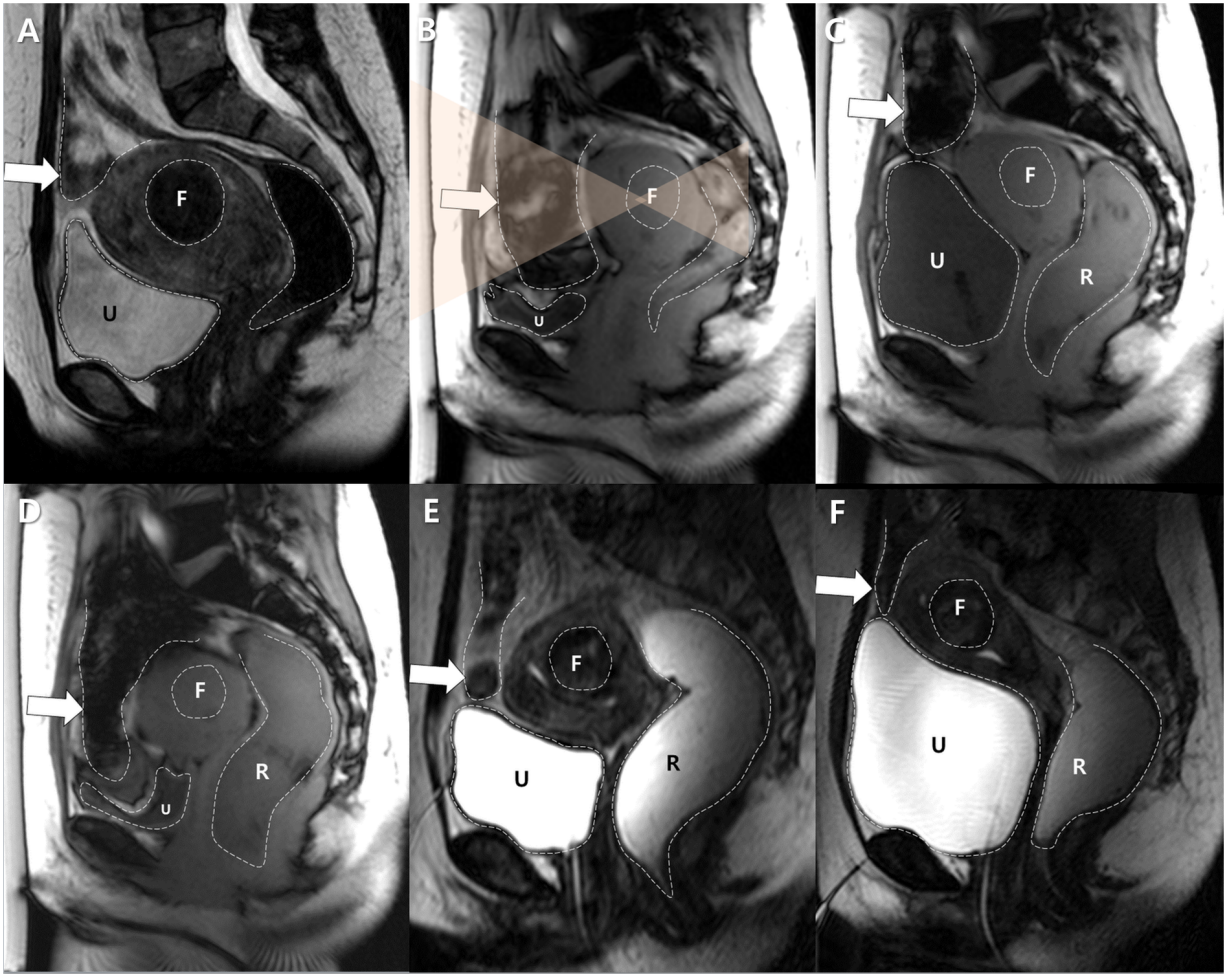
A case of technical failure of the BRB maneuver in a 42 year-old woman with a fibroid in a forward-bent uterus. The patient did not undergo GnRH agonist pretreatment. A. Sagittal image of screening MRI showed a uterine fibroid of the submucosal type (F). The uterus was bent forward and measured 108 mm in its largest dimension. The bowel loops were interposed. F indicates the target fibroid and U indicates the urine-filled urinary bladder. Dotted lines delineate the margins of each structure. B. On treatment day, a sagittal image from the survey scan revealed that more bowel loops (arrow) were interposed and the uterus shrank to 68 mm for an unknown reason. F and U indicate the target fibroid and the urinary bladder, respectively. Dotted lines delineate the margins of each structure, and transparent orange triangles represent the planned HIFU beam path. C. The urinary bladder (U) was filled, followed by the rectum (R). However, the bowels (arrow) were located within the anticipated sonication path, even after transducer angulation. Moreover, the target fibroid (F) was too deeply located to allow a safe and effective ablation. Dotted lines delineate the margins of each structure. D. After emptying the urinary bladder (U), the bowel loops (arrow) descended again completely blocking the target fibroid (F). R indicates the rectum. Dotted lines delineate the margins of each structure. E. The urinary bladder (U) was filled with saline (300 mL) again. However, there was still a bowel loop anterior to the uterus. Urinary bladder filling and emptying was repeated 5 times in this particular case. F and R indicate the target fibroid and the rectum, respectively. Dotted lines delineate the margins of each structure. F. Further filling of the urinary bladder (U) with saline (500 mL in total) and the rectum (R) with gel (350 mL in total) did not satisfactorily displace the interposed bowel loop (arrow). Therefore, the procedure was terminated without initiation of MR-HIFU sonication. Dotted lines delineate the margins of each structure.
